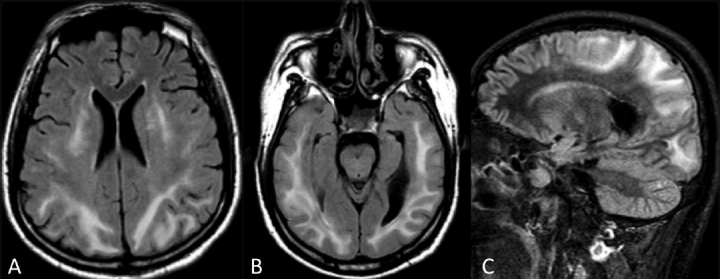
MRI of the brain in June, Axial FLAIR (A,B) and sagittal STIR-FLAIR (C) images show bilateral signal hyperintensity of the supratentorial white matter of the brain. The signal abnormalities have a predilection for the territories of the posterior circulation
